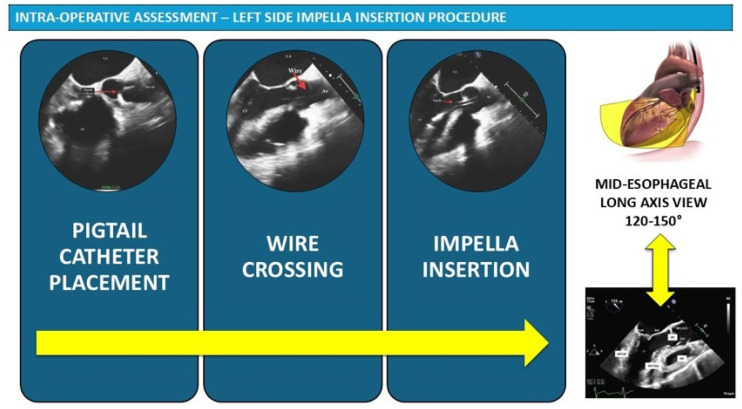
Main views and steps for intra-operative echocardiographic assessment for Impella^®^ positioning. 3-steps approach for left side Impella positioning: the mid-esophageal long-axis view is pivotal in evaluating the proper positioning and the possible interference of the Impella catheter with mitral valves and sub-valvular apparatus.
